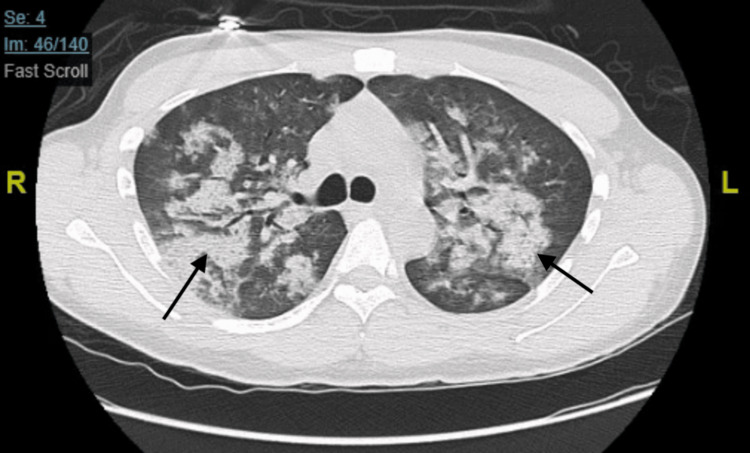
CT chest with contrast showing multiple diffuse ground-glass opacities. Black arrows showing ground-glass opacities.
